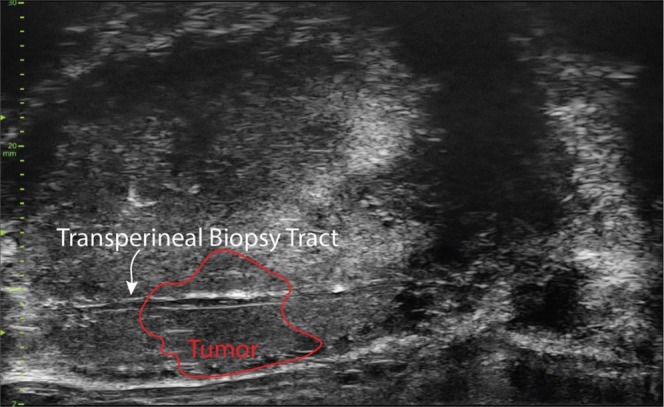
Micro‐ultrasound image with a biopsy tract following tissue removal with an 18‐gauge biopsy needle. Imaging parameters of all tissue samples were recorded via a cine sweep of the post‐biopsy tissue. For example, this target bounded in red was considered isoechoic.
